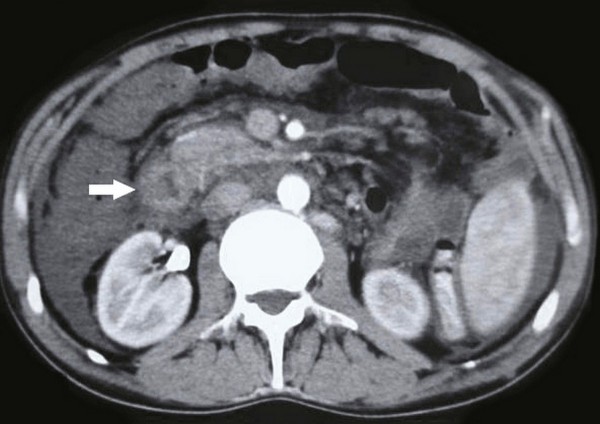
Late phase contrast enhanced CT without oral contrast demonstrates diffuse wall thickening and narrowing in second part of the duodenum (arrow).
